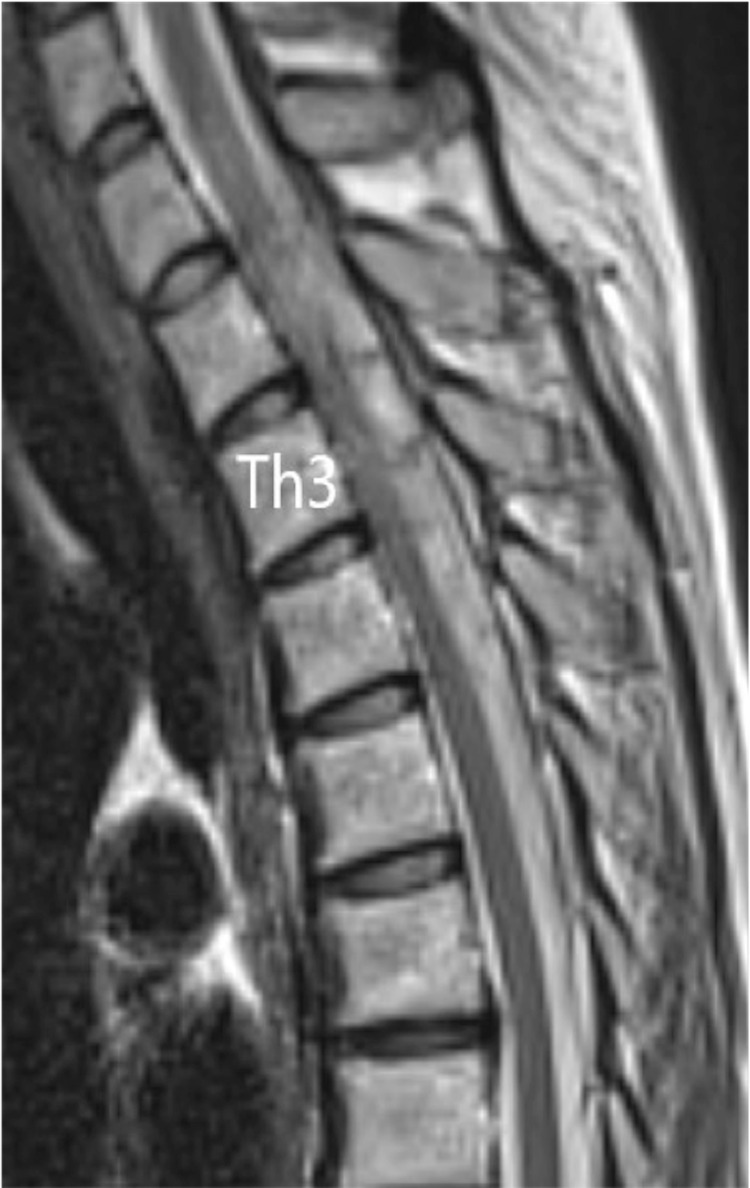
Sagittal T2-weighted MR images at the time of transfer In the T2-weighted image, the signal was higher than that of the spinal cord and lower than that of the cerebrospinal fluid.
